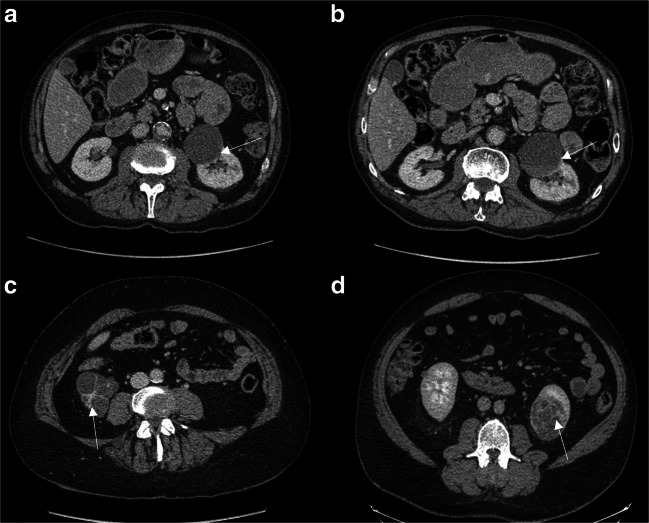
Post-contrast axial CT images of complex left renal cysts. a Bosniak IIF cyst progressing to (b) Bosniak IV after 6 months with progressing enhancing wall (arrows), clear cell carcinoma, pT1a grade 2. c Bosniak III cyst with thick enhancing septae (arrow), clear cell carcinoma, pt1a grade 1. d Bosniak IV cyst with thick septa (arrow), clear cell carcinoma, pT1b grade 2
